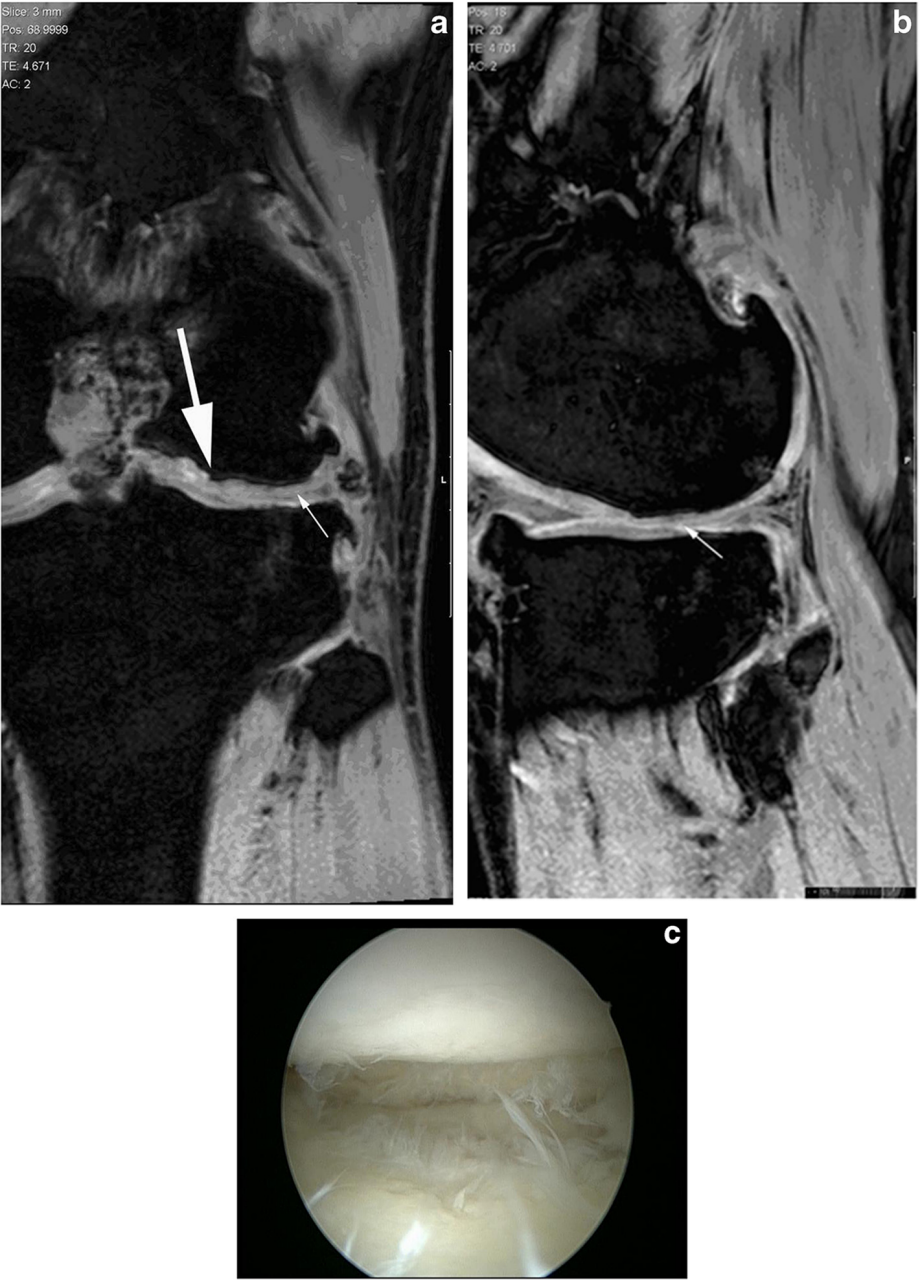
Second year follow-up magnetic resonance imaging. (a) Coronal and (b) sagittal three-dimensional gradient echo T1 (WATSf; repetition time 20, echo time 5.2, field of view 150, fractional anisotropy 15°, slice thickness 3 mm) left knee magnetic resonance images demonstrate the morphology of the cartilage implant. The graft is well integrated with a thickness that is similar to that of the adjacent cartilage; the graft surface is smooth (arrow). Mildly decreased signal intensity and artifacts are observed in the graft compared with the adjacent cartilage. Underneath, the large graft irregular bone contour (thick arrow) and osteophyte formation at bony margins is also noted. (c) Second-look arthroscopy at two years demonstrated complete filling of the defect and excellent integration of the newly formed cartilage with native tissue.
